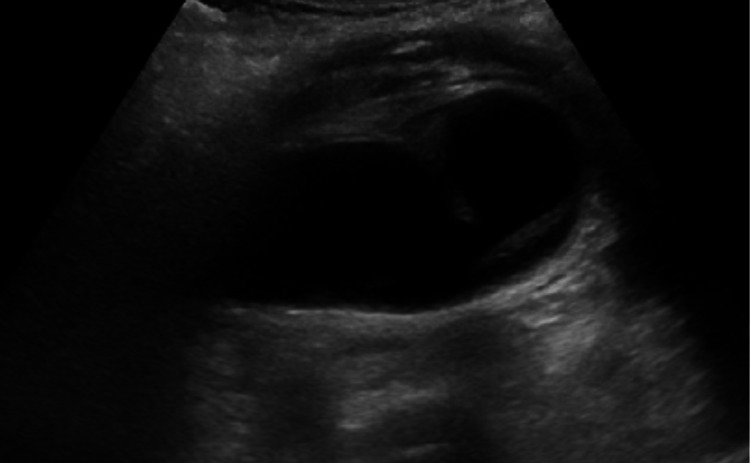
Abdominal ultrasound. Abdominal ultrasound revealed a distended gallbladder with possible internal debris and suggestion of wall thickening. No stones were visualized.
